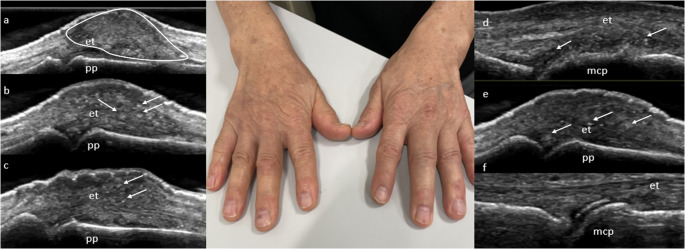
Ultrasound in the differential diagnosis of psoriatic arthritis. Legend. A man was referred to rheumatology with polyarticular joint pain and swelling. He had a previous diagnosis of gout (serum urate <400 µmol/L on allopurinol 300 mg/day) and a family history of PsO, raising suspicion for PsA. No cutaneous tophi were present. (a–c, d) Dorsal longitudinal images of the metacarpophalangeal region showing hyperechoic deposits within and adjacent to the extensor tendon and over the proximal phalanx, consistent with monosodium urate (MSU) crystal deposition, including cloud-like deposits (outlined in white in figure a) and small urate aggregates, which are pointed out by arrows in b and c. Dorsal longitudinal images of the metacarpophalangeal joints demonstrate intra-synovial MSU crystal aggregates (arrows in d), intra-tendinous and peri-tendinous deposits (e) and crystal deposition along the cartilage surface consistent with a double contour sign (f). Acronyms: et: extensor tendon; mcp: metacarpophalangeal; pp: proximal phalanx
